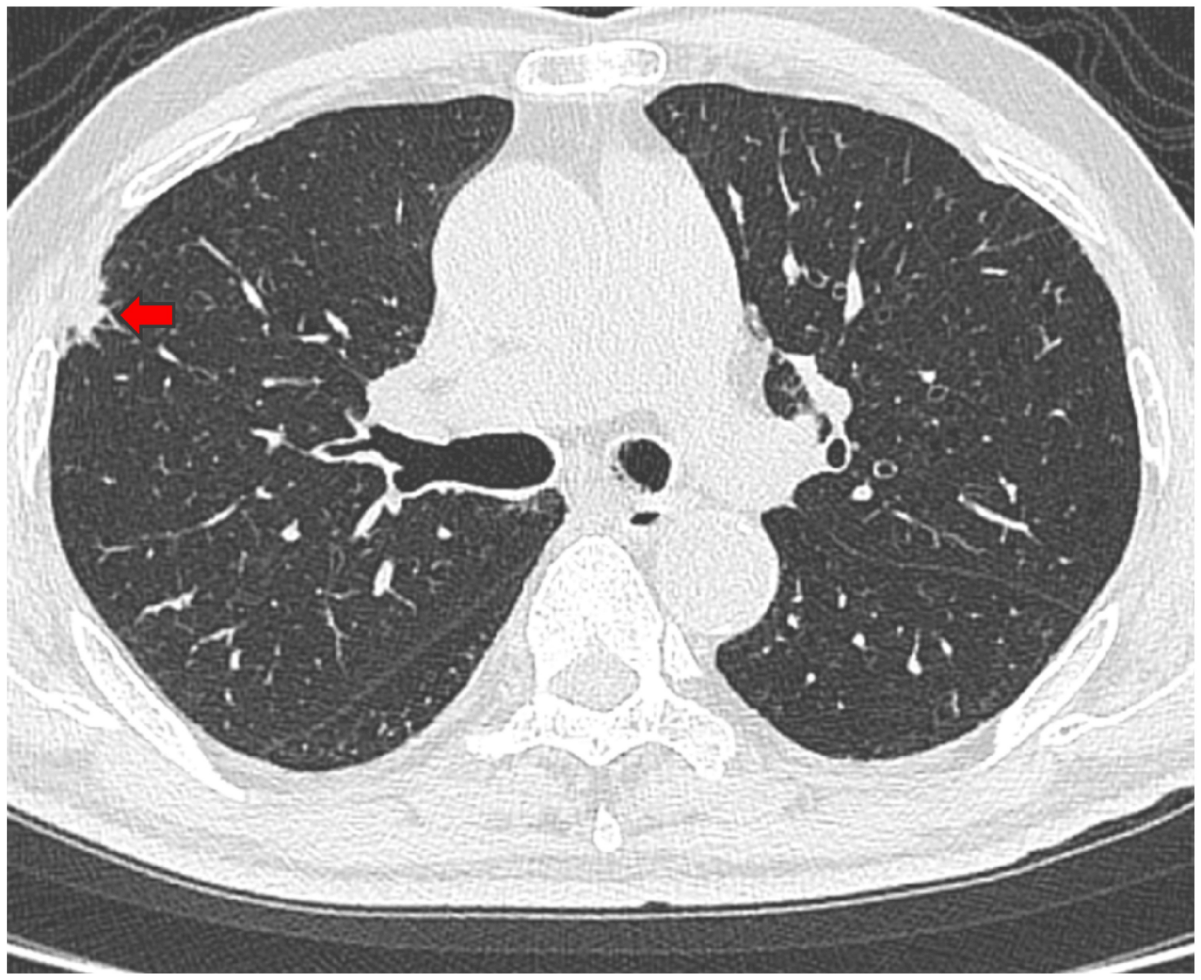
CT findings at the initial and last visits for Case 1. A 12 × 9 mm nodule was found in the right upper lobe. The arrow denoted the nodule.
